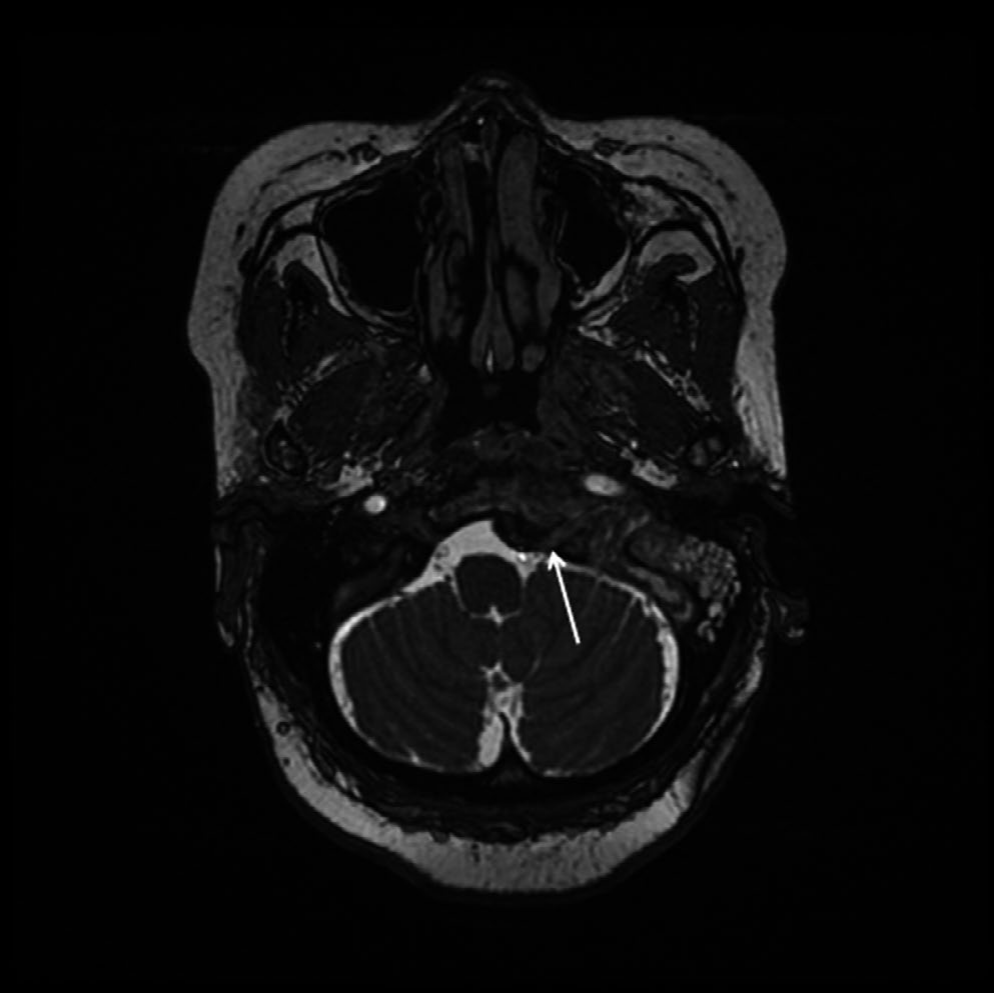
T2‐weighted thin‐slice FIESTA MRI sequence shows a thickened left hypoglossal nerve (open arrow) within hypoglossal canal narrowed by inferior aspect of mass
